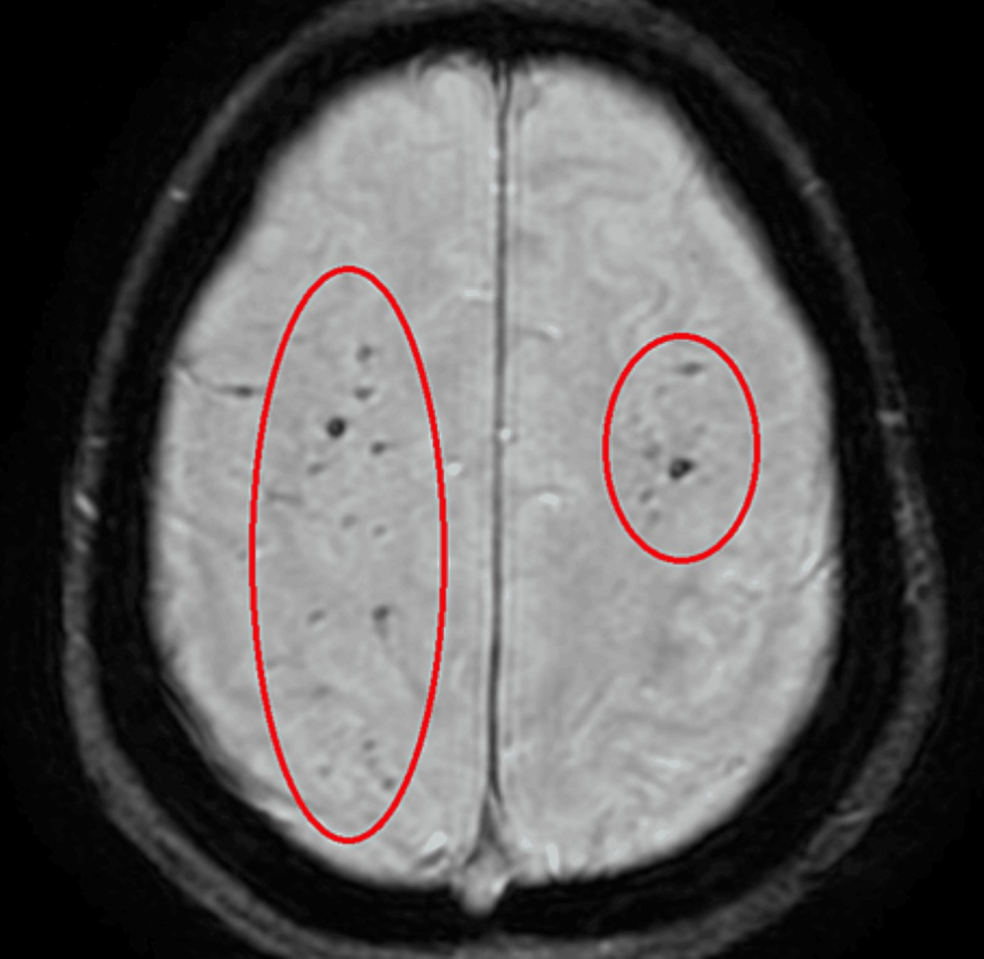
Susceptibility-weighted angiography (SWAN) sequence of the MRI showing the sequela of previous microhaemorrhages.
